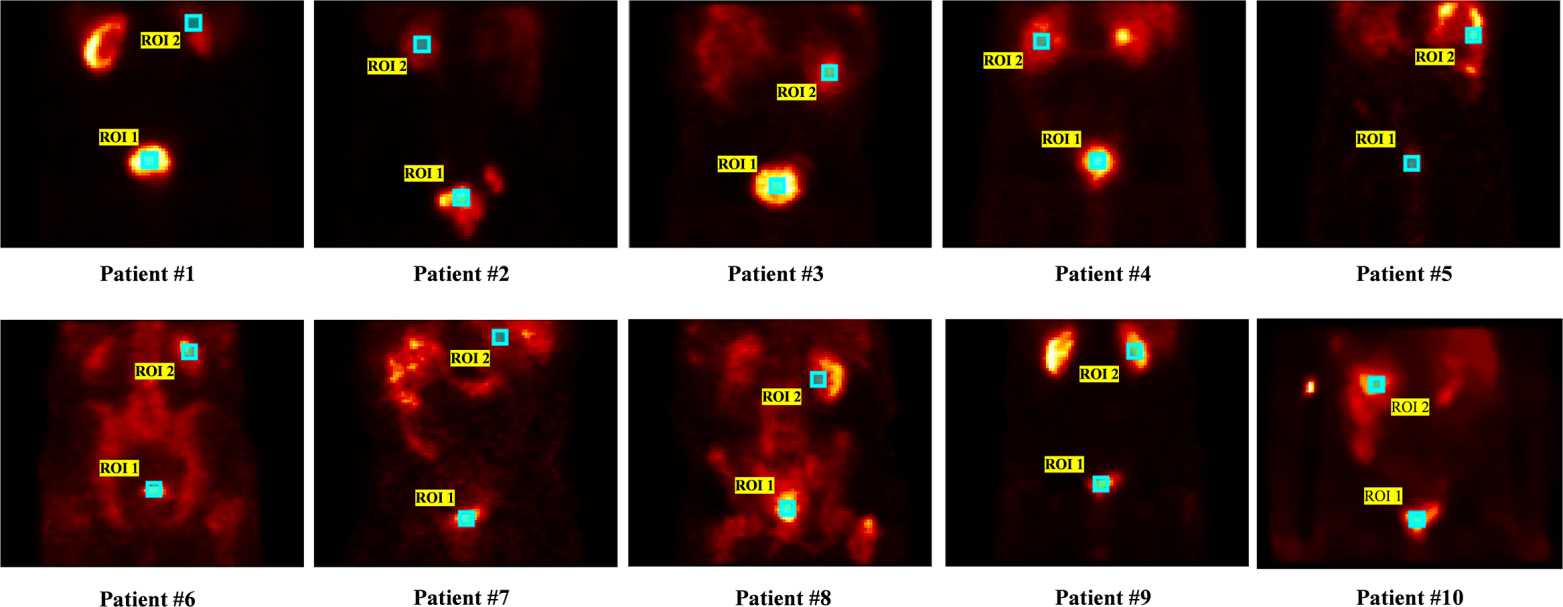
ROIs were drawn on the SPECT images of 10 patients: ROI1 (prostate lesion), ROI2 (physiologic reference-renal cortex). ROIs, region of Interest; SPECT, single photon emission computed tomography.
